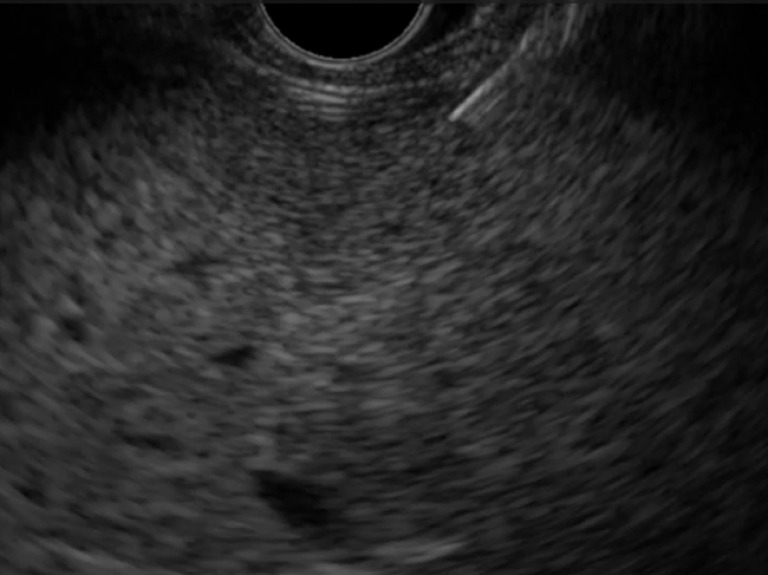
The puncture needle is not visible during endoscopic ultrasound-guided liver tissue acquisition. Subsequently, it was found that fixation of the high frequency transducer was loose and rotated 10° counterclockwise.
